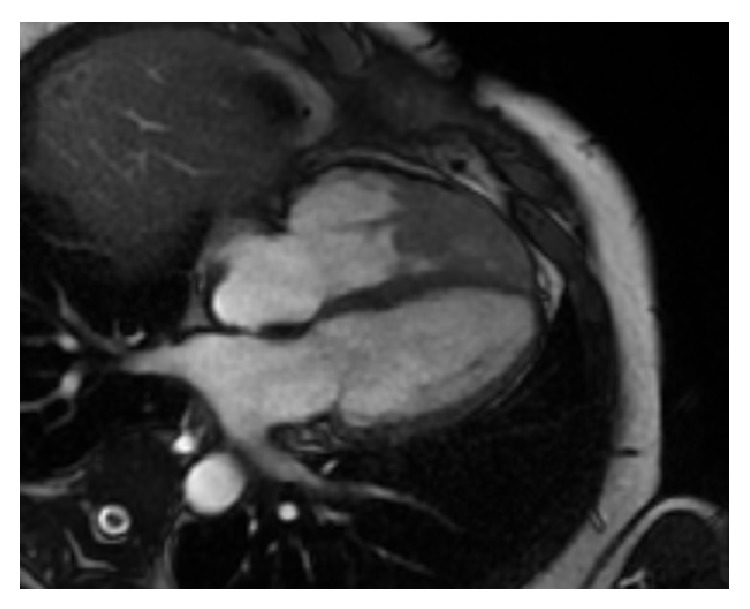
Cardiac MRI showing RV mass. On MRI, the mass was found to involve the apex and mid right ventricle. Ejection fraction on the left ventricle was estimated to 60%. There was also noted turbulence over the aortic valve, suggestive of a possible mass.
